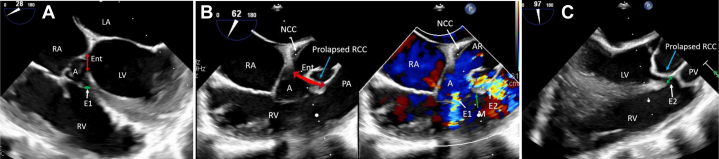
TEE Views of a Complex pmVSD Type (A) Four-chamber view shows a large left-sided entrance (Ent, red double-headed arrow) and a small posteroinferior exit (E1, green double-headed arrow) associated with inlet extension and septal aneurysm (A). (B) Modified short-axis view shows the entrance extending beneath the NCC and the RCC, with RCC prolapse partially covering the defect (blue arrow). On the RV side, the defect divides into 2 exit points: E1 located posteroinferiorly associated with aneurysm (A), and E2 located antero-superiorly just beneath the pulmonary valve (PV) and the PA. Muscular separation (M, green arrow) is visible between the 2 exit points. (C) Modified long-axis view shows the anterosuperior exit point (E2: green double-headed arrow) more clearly. This case is a combination of echocardiographic types A and D and was successfully closed by 2 devices (MFO for E1, fixed inside the aneurysm, ADO II for E2). LA = left atrium; other abbreviations as in Figure 1, Figure 2, and 5.
